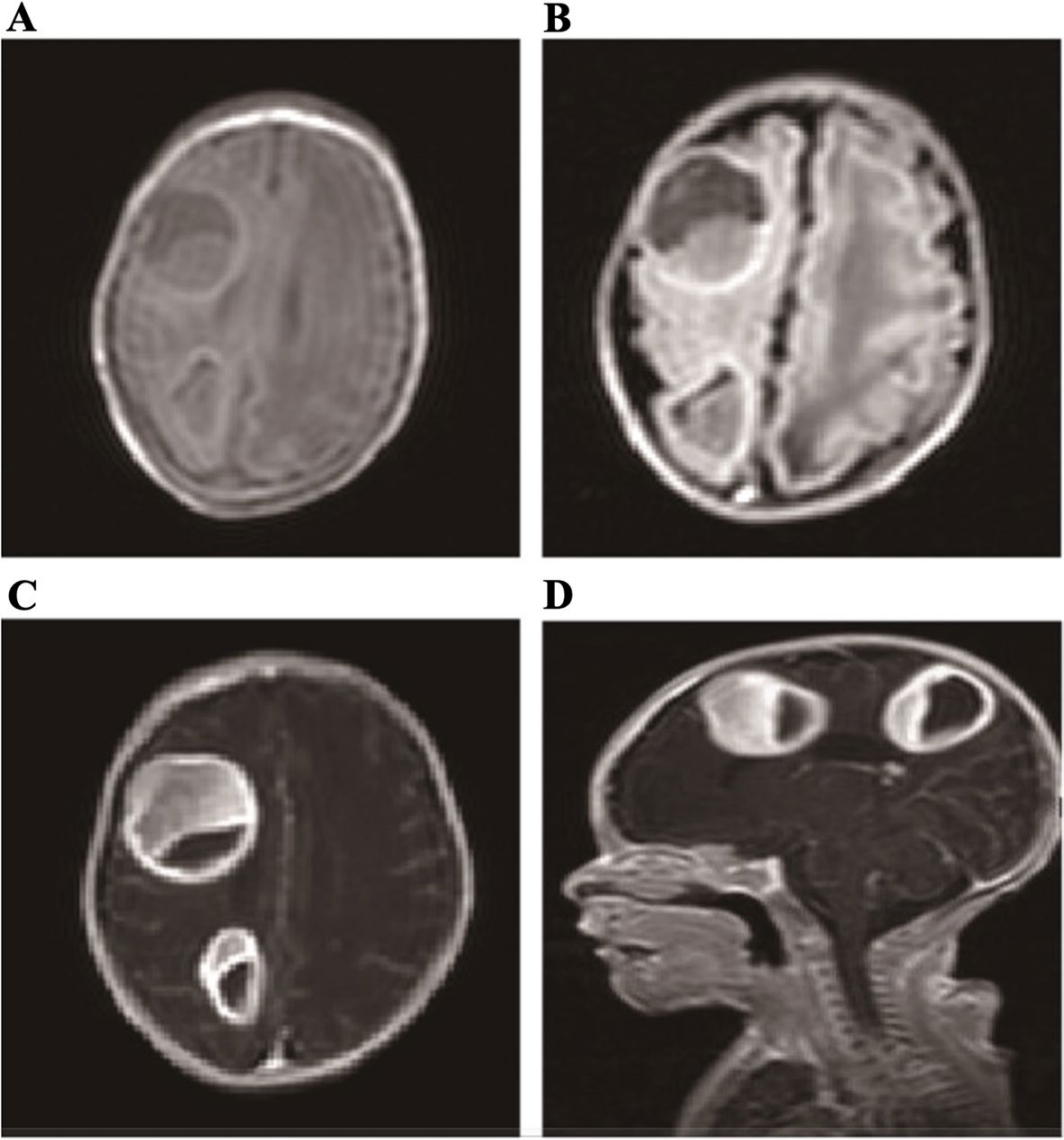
The brain revealed multiple space-occupying masses in the frontal and parietal lobes in the right brain. A and B showed the T1-weighted MRI image and T2-weighted MRI image respectively. C and D showed the T1-weighted contrast-enhanced MRI scan of the brain obtained after administration of an intravenous contrast agent
